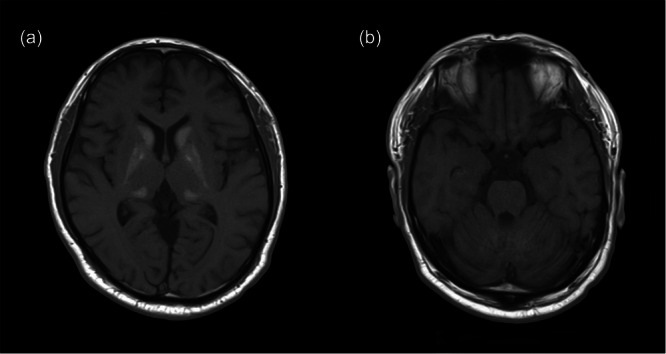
Brain magnetic resonance imaging findings. Fluid‐attenuated inversion recovery (FLAIR) images show (a) symmetrical hypointense lesions corresponding to calcifications in the bilateral basal ganglia, thalami, and cerebellar dentate nuclei, consistent with chronic intracranial calcifications related to idiopathic hypoparathyroidism and (b) no hippocampal atrophy or abnormal signal intensity.
